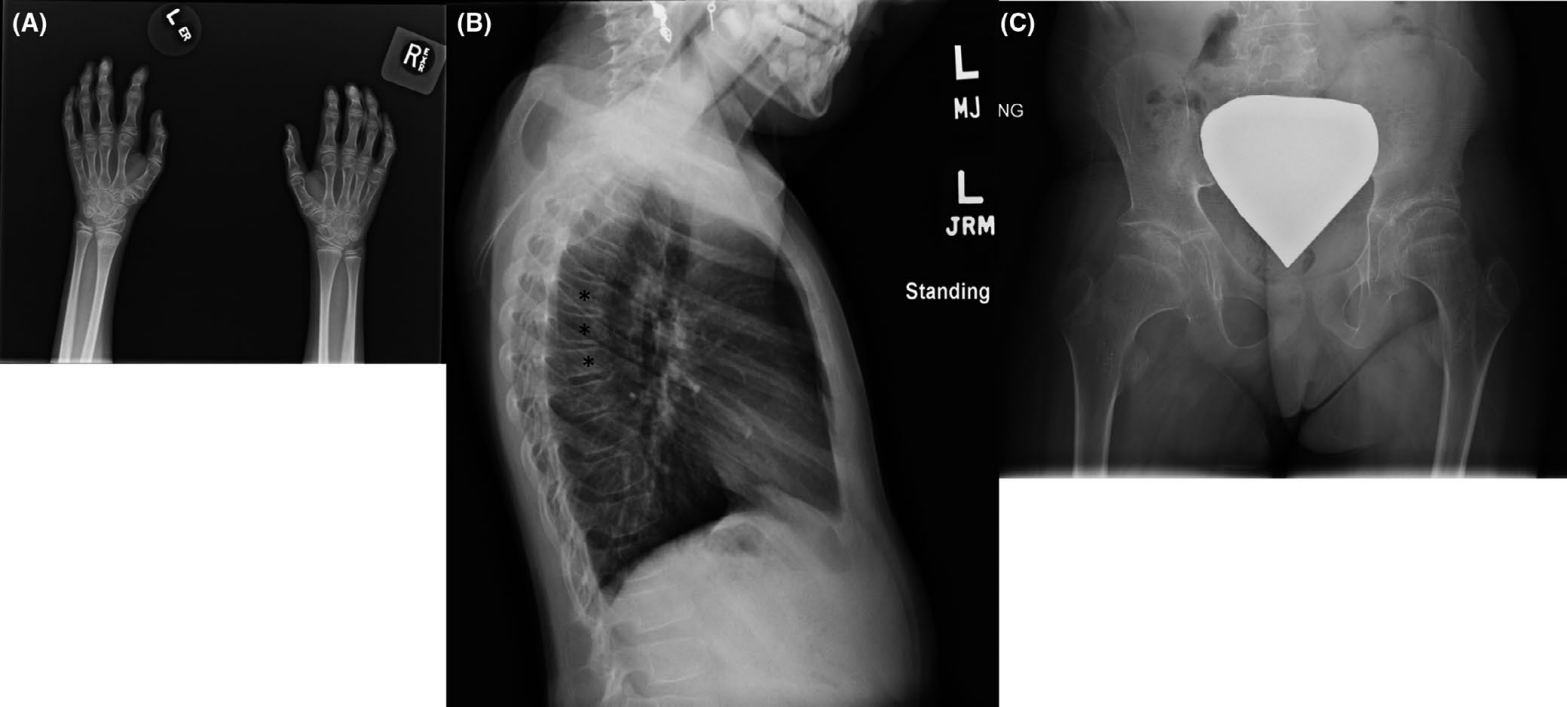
Radiograph of hands show the phalanges are shorter and broader, diffuse osteopenia, and narrowing of joint space in the wrists (A), spine shows platyspondyly indicated by asterisks (B) and hips show joint space narrowing, osteopenia, and erosion of the femoral heads (C)
